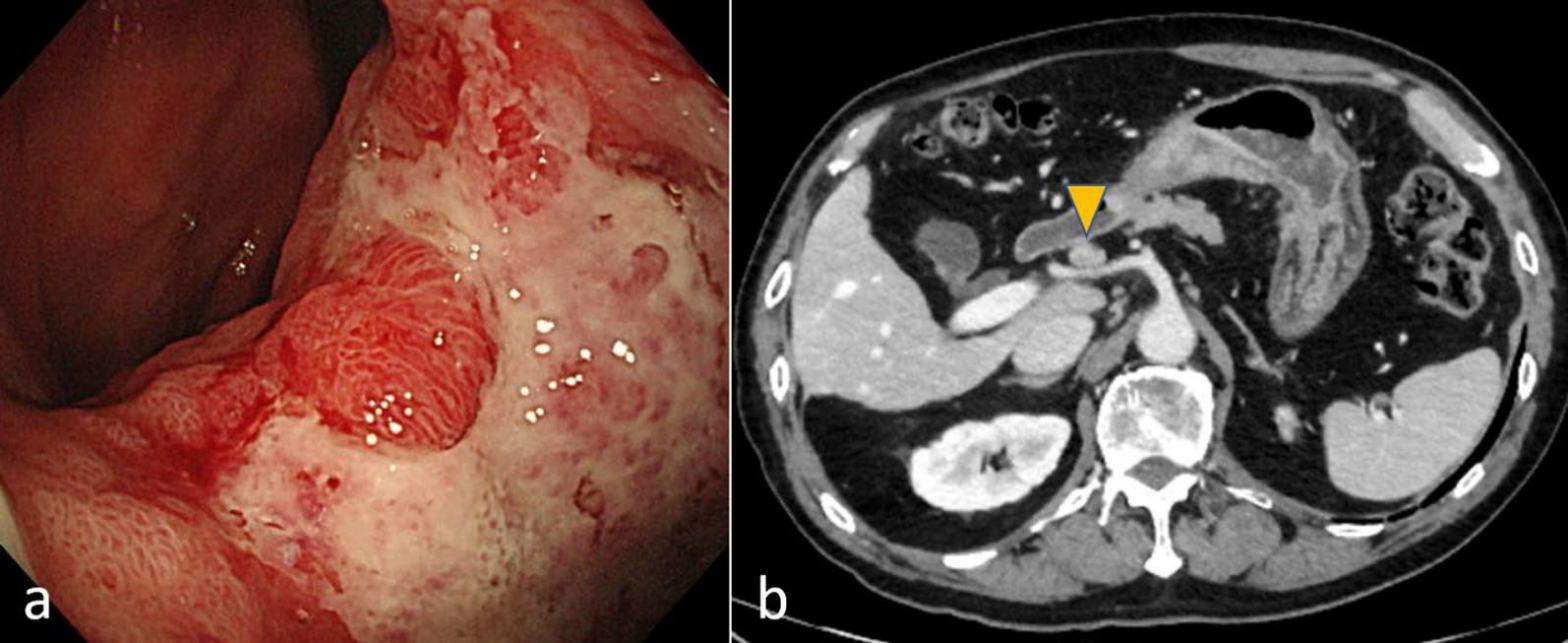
Findings of case 1 with gastrointestinal endoscopy and abdominal computed tomography before treatment. a A type 3 gastric cancer in the lesser curvature extending to the posterior wall of the middle to lower gastric body was found. b The station No. 8a lymph node (arrowhead) was enlarged
